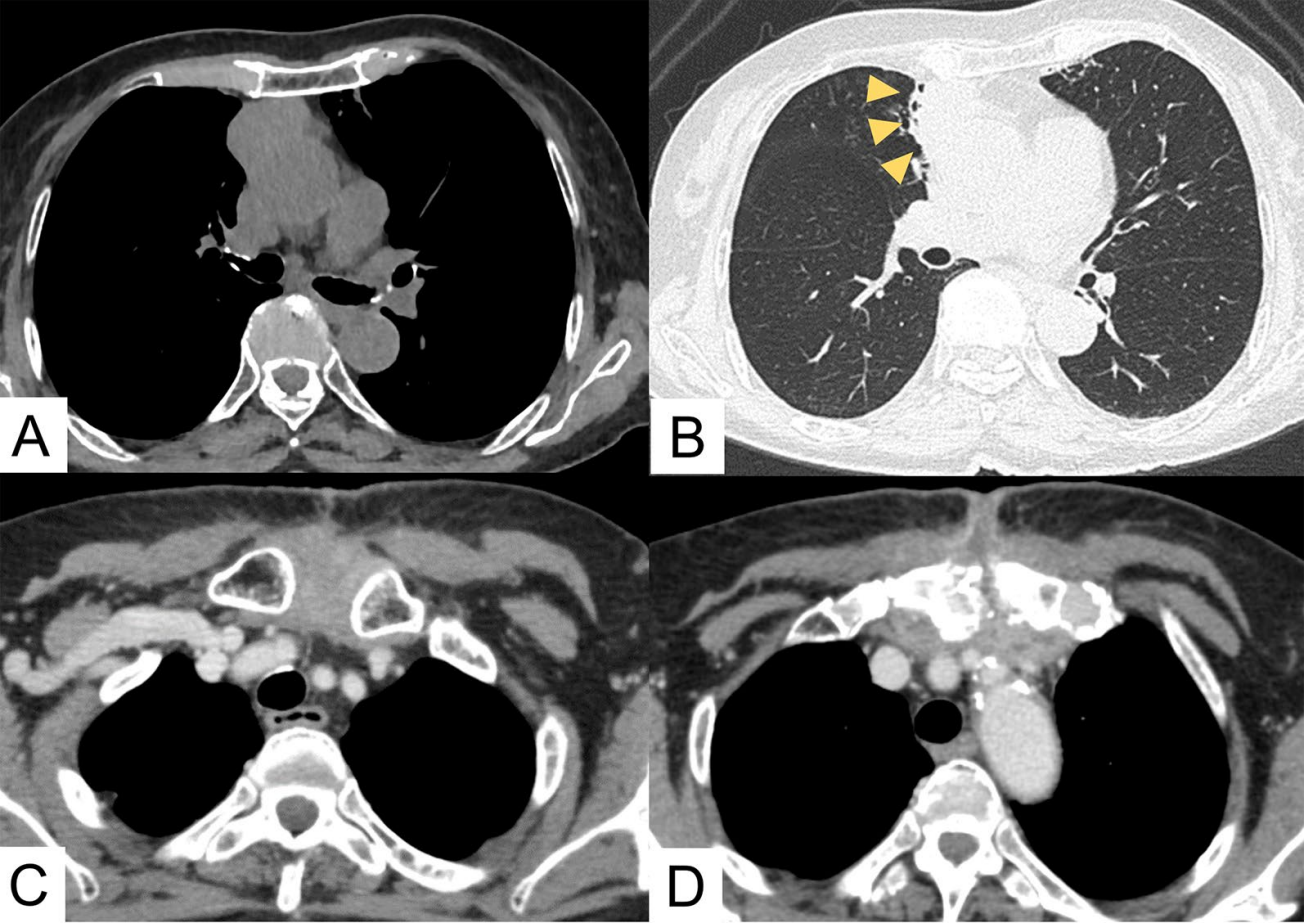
A, B Preoperative CT shows anterior mediastinal tumor adjacent to inflammatory changes in right upper lobe (yellow arrowheads). C, D CT before surgical drainage revealed abscess spreading around sternal notch to manubrium, and focal mediastinal thickening
